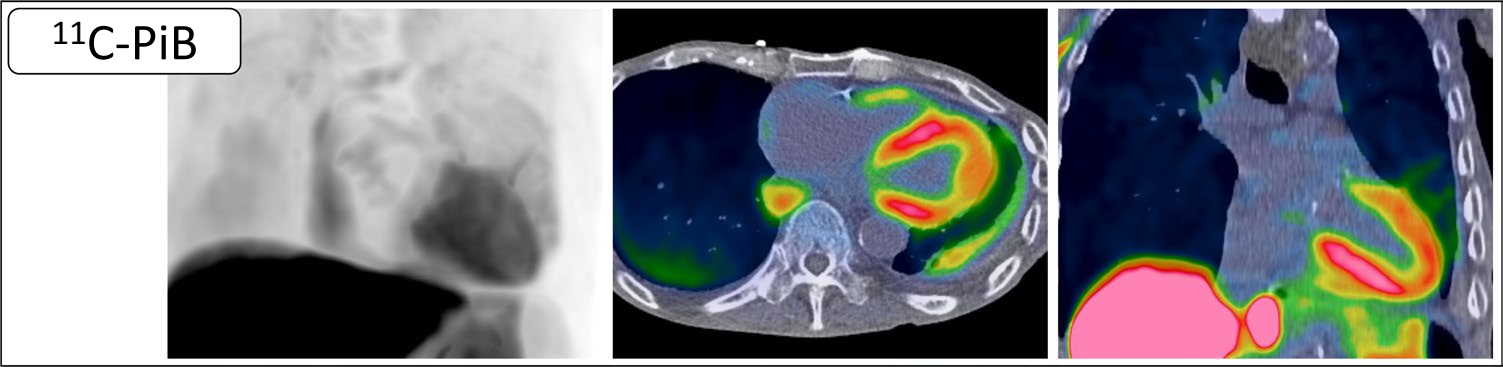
Representative case of AL cardiac amyloidosis evaluated with ^11^C-Pittsburgh compound B (PiB) PET. A male patient in his 70 s was diagnosed with AL cardiac amyloidosis based on biopsy findings. Shown from left to right are maximum intensity projection (MIP), axial PET/CT, and coronal PET/CT images. Diffuse radiotracer uptake is evident in both the left and right ventricular myocardium, with mild uptake also observed in the atrial walls
